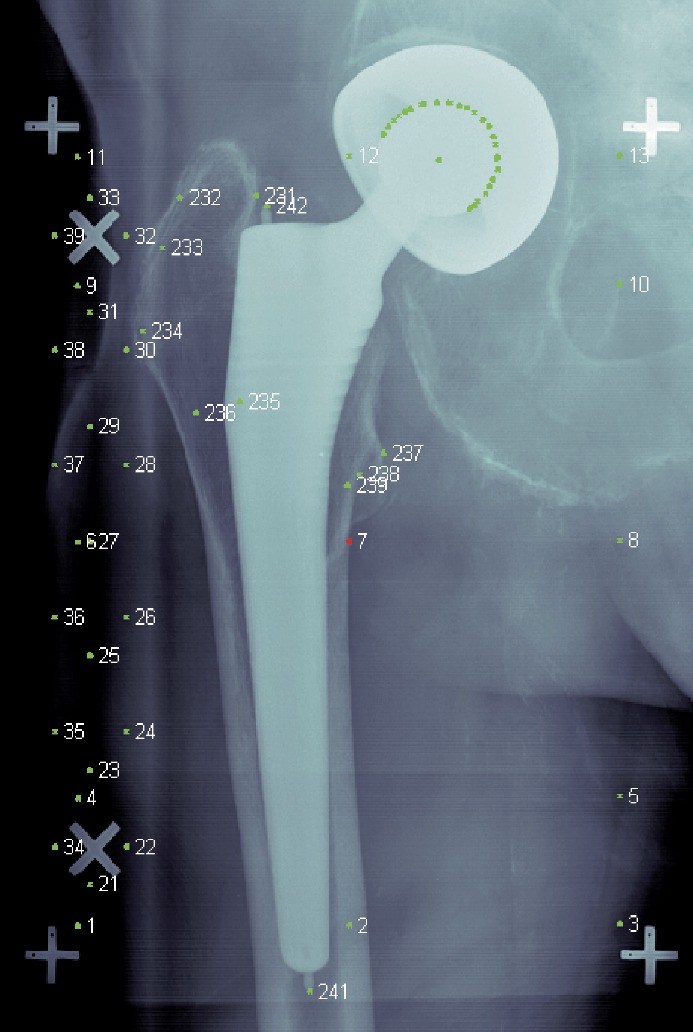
UmRSA radiograph of the Corail stem with a bi-articular Monk head. About 8 tantalum beads, 0.8 mm in diameter, were inserted in the bone (nos. 232–239). A tantalum bead of 0.8 mm in diameter was mounted on the tip of the stem (241) and at the shoulder of the stem (242). These 2 beads and the head were used to calculate stem configuration. In the case of hemiarthroplasty, the 26-mm head inside the Monk bi-articular head was visible and used. Cage markers: 1–40.
